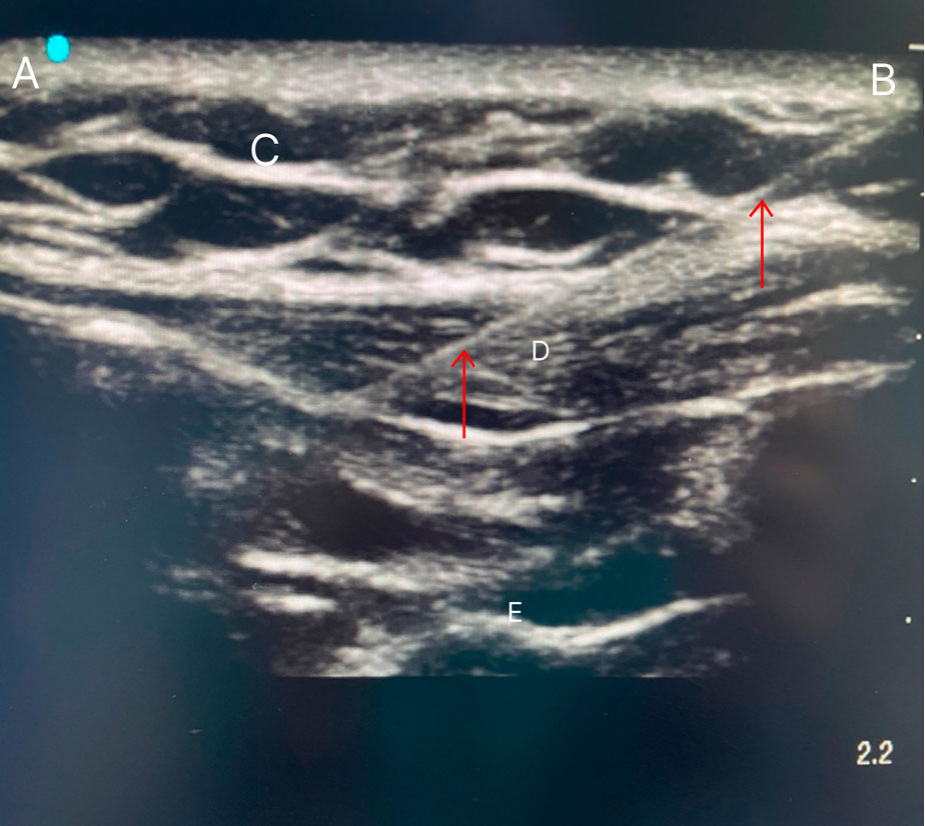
Ultrasonographic image of EOI. A, Medial aspect; B, lateral aspect; C, subcutaneous fat; needle (red arrows); D, EO; E, pleura.
